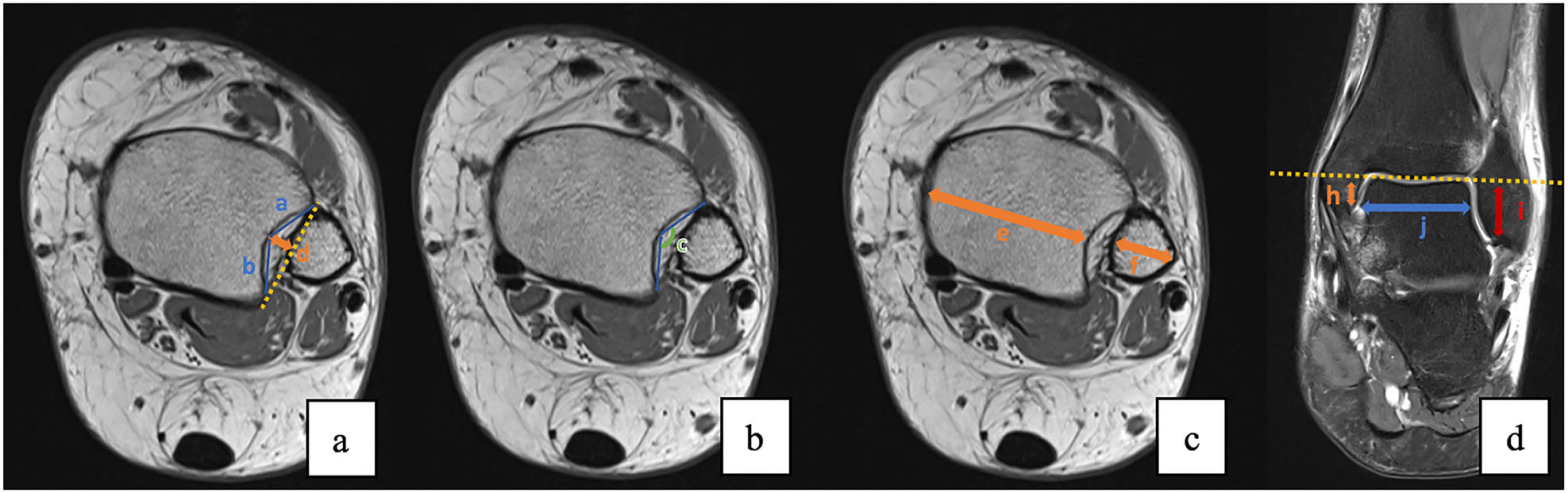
MRI T1-weighted images on the axial plane: a–c. MRI T2-weighted image on the coronal plane d. Representation of the measurements made on MRI sections
